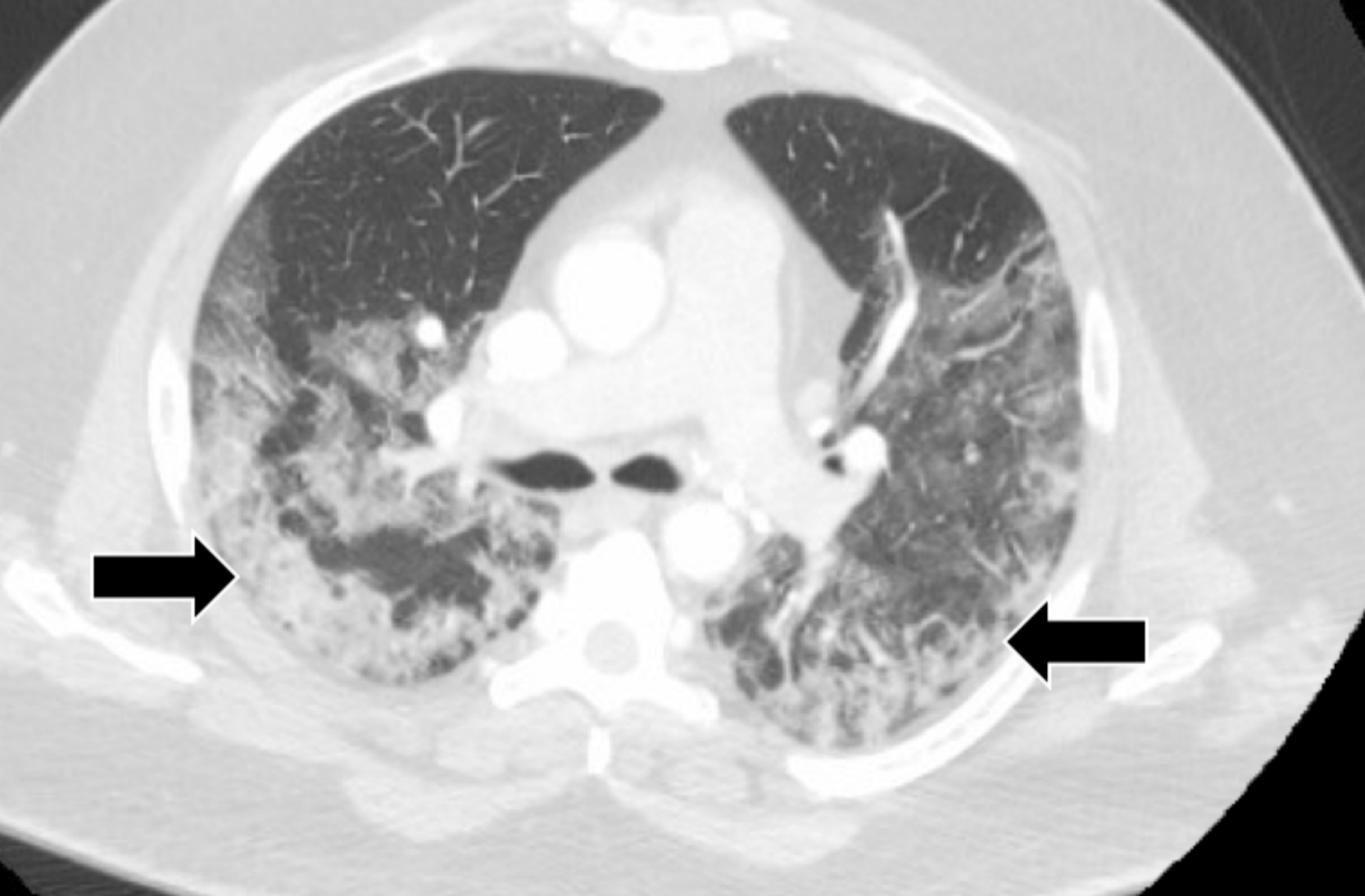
CT chest showed bilateral ground-glass opacification CT: computed tomography
